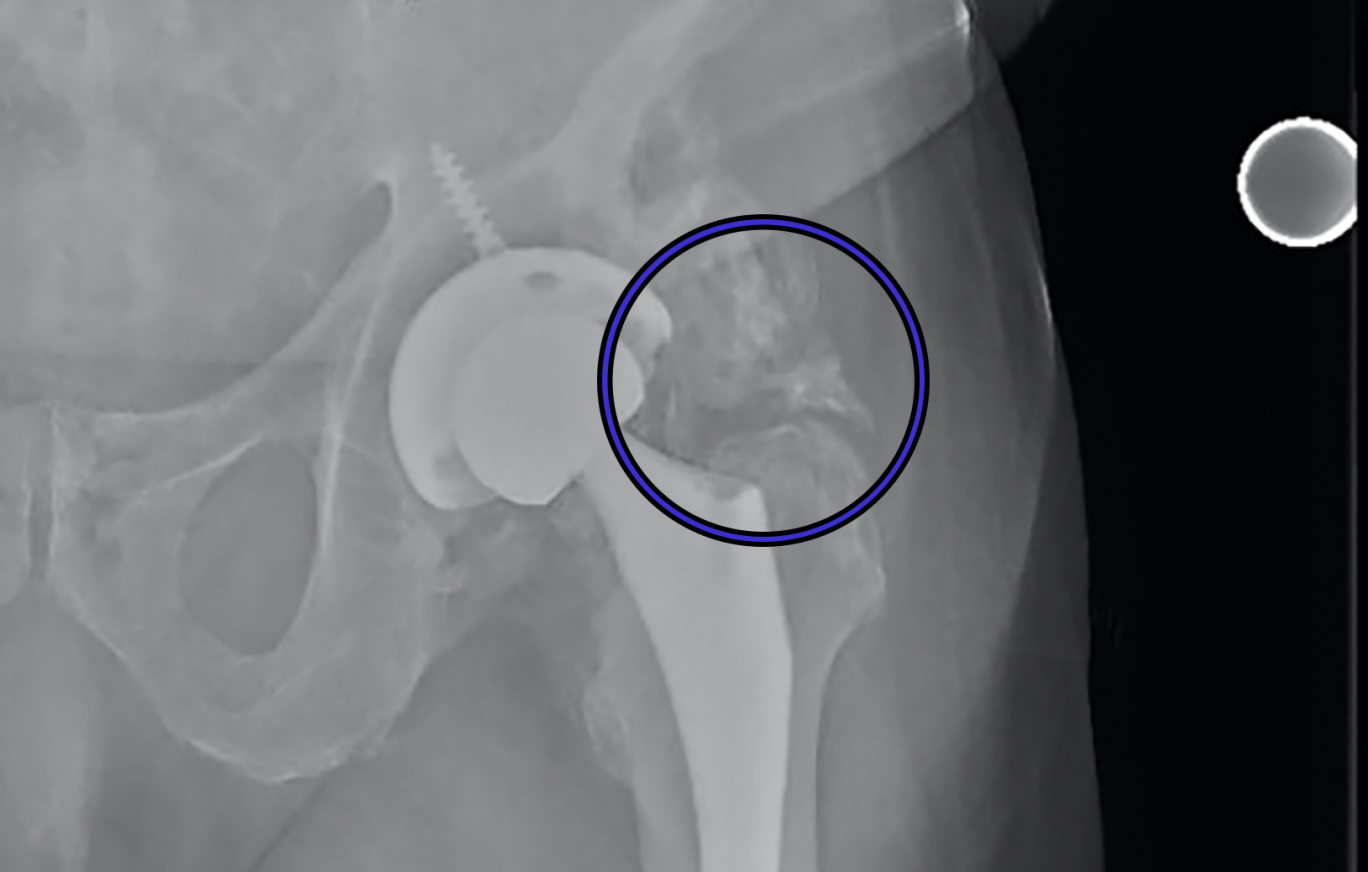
Anteroposterior (AP) radiographs of post-op total hip arthroplasties with differing classes of HO according to the Brooker classification Class 3: bone growth extending from femur and pelvis with <1cm between ends
